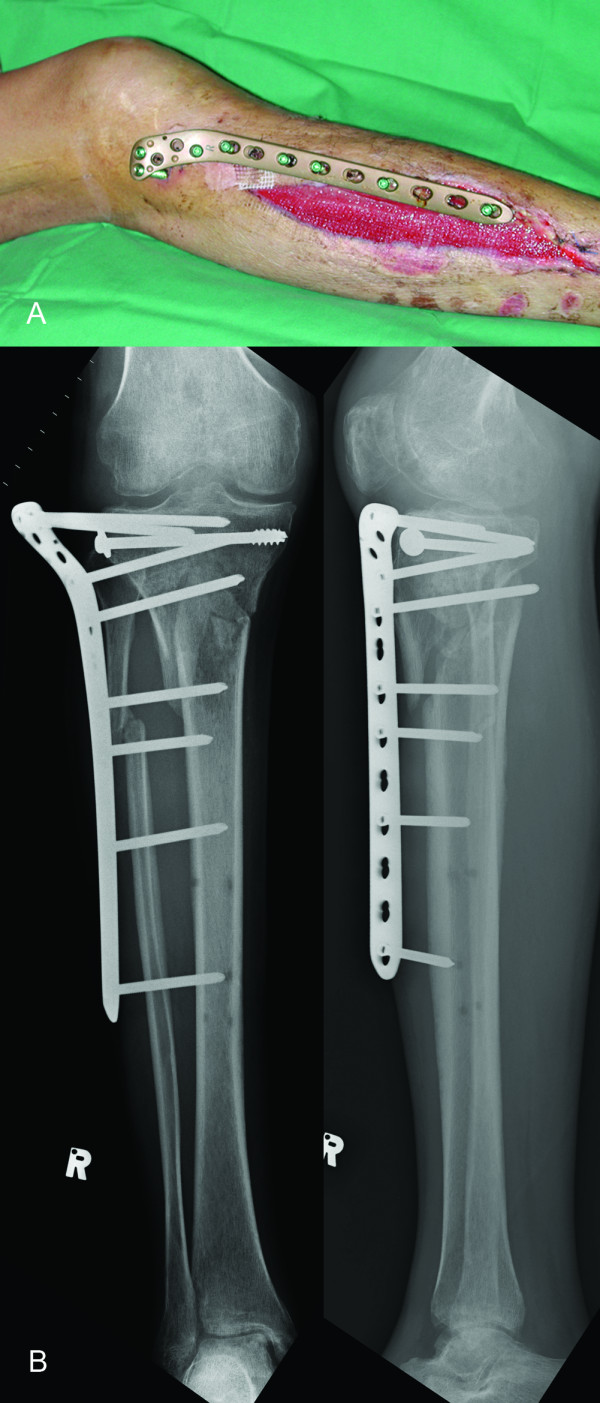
a - External appearance of proximal tibia LCP applied as an external fixator. b - Postoperative radiograph showing proximal tibia LCP external fixation.
